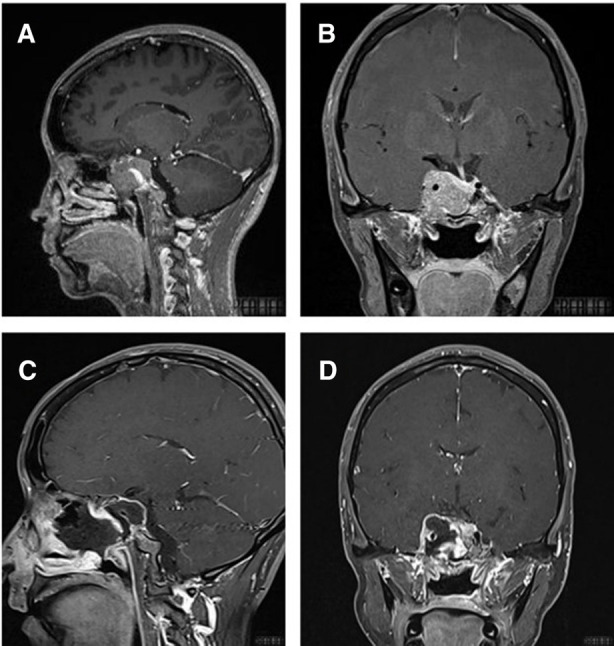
T1-weighted MRI image with contrast enhancement of a cavernous sinus pituitary tumor. (A) Preoperative sagittal view. (B) Preoperative coronal view. (C) Postoperative sagittal view. (D) Postoperative coronal view. Note in (C) and (D) the autologous fat employed intradurally to fill empty space within the sellar after tumor removal.
